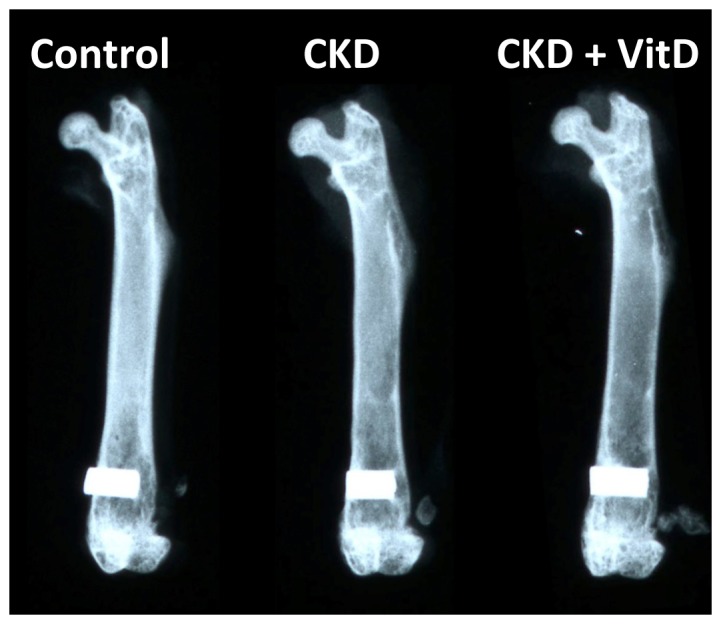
X-ray examination of the femurs after 2-weeks healing. The implants were surrounded with bone without any notable radiotranslucent gap.
